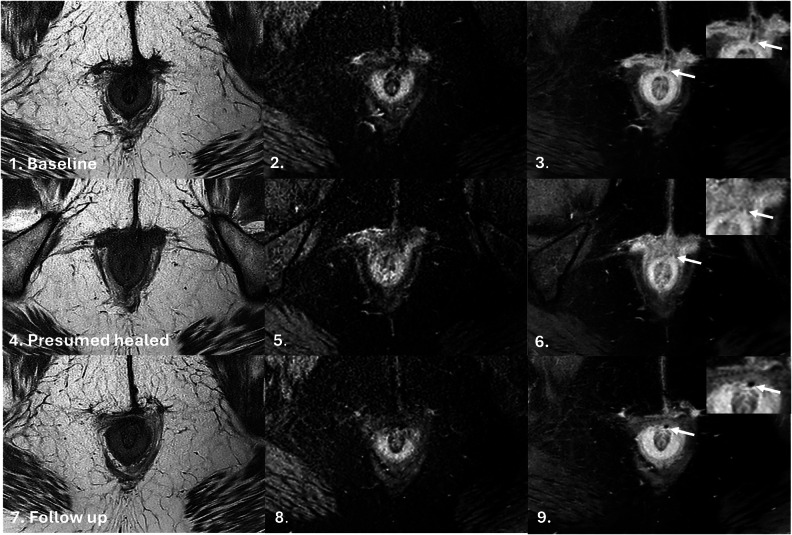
TOpClass-radiologically healed fistula with clinical recurrence. From top to bottom, left to right: Baseline MRI. 1 Axial oblique T2-weighted, (2) fat-saturated T2-weighted and (3) post-contrast fat-saturated T1-weighted images show subtle hyperintensity (arrow) with a central hypointense seton, consistent with ongoing fistula activity. Presumed healed MRI (4–6) shows a hypointense tract at the previous fistula site on all three axial oblique sequences, consistent with fibrosis and absence of overt active inflammation, meeting criteria for radiological healing. Follow-up MRI (7–9) (6 months later) demonstrates re-emergence of a hyperintense tract at the same site (arrow), consistent with recurrent fistula activity
